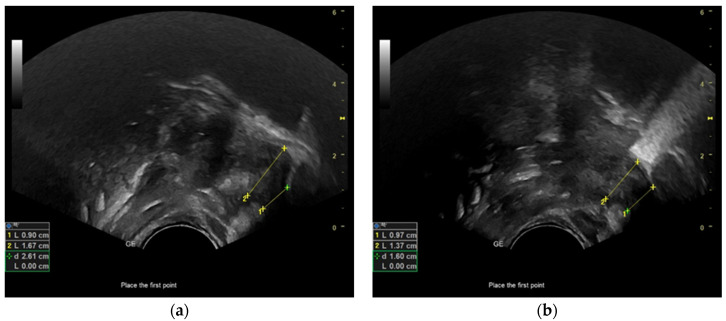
Ultrasonographic image of the tongue during breastfeeding: (a) tongue up; anterior tongue height, mm: yellow line 1; posterior tongue height, mm: yellow line 2; (b) tongue down; anterior tongue height, cm: yellow line 1; posterior tongue height, cm: yellow line 2.
